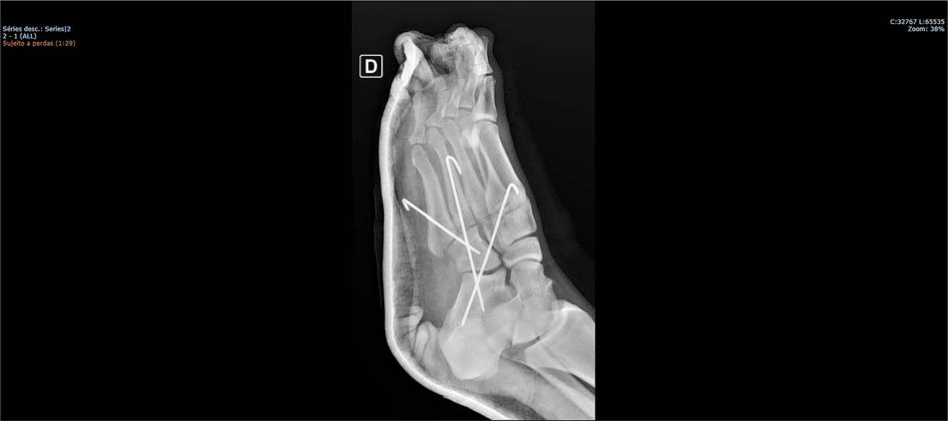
Postoperative X-ray of the foot in lateral view
